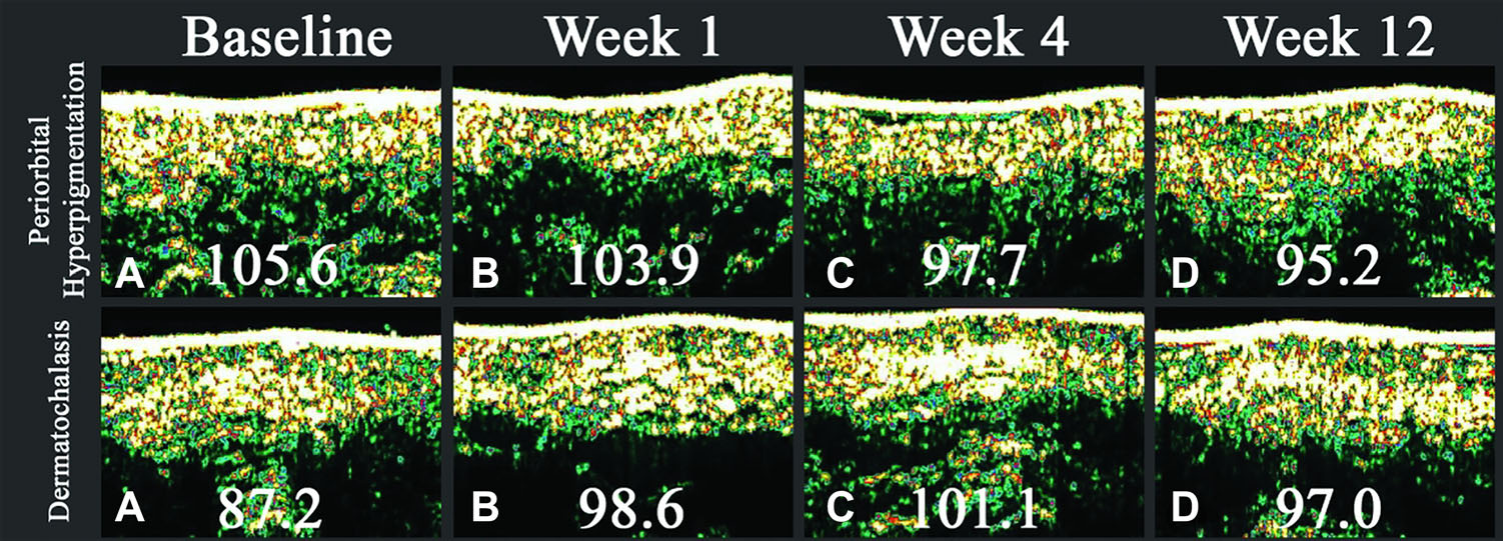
Image produced from the ultrasound probe of DermaLab® Combo for the same periorbital hyperpigmentation and dermatochalasis patients in Fig. 2 and 2, A before receiving treatment, B 1 week after injection, C 4 weeks after injection, and D 12 weeks after injection. Intensity scores (of arbitrary units, indicating ultrasound signal intensity) are shown for each study. Though the calculated intensity score had decreased throughout the 12 weeks for the periorbital hyperpigmentation patient, signal intensity seems relatively stable, and the thickness of the dermis did not change substantially. On the other hand, signal intensity had increased noticeably for the dermatochalasis patient, with mild tapering of effects at week 12. Increased thickness of the dermis is also observed
